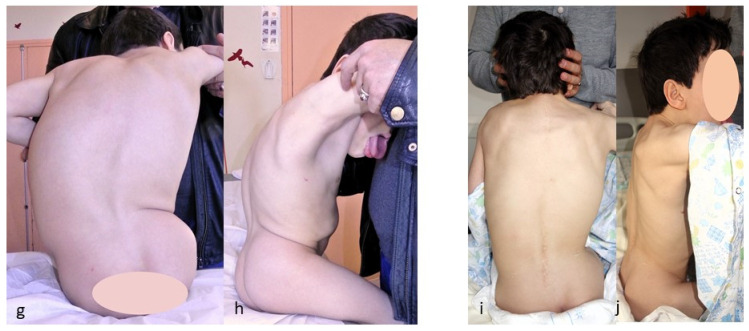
(a,b) Preoperative radiographs of a 12-year-old boy with cerebral palsy; (c,d) postoperative radiographs; (e,f) Radiographs after rod lengthening; (g–j) initial and 6-year postoperative clinical pictures.
